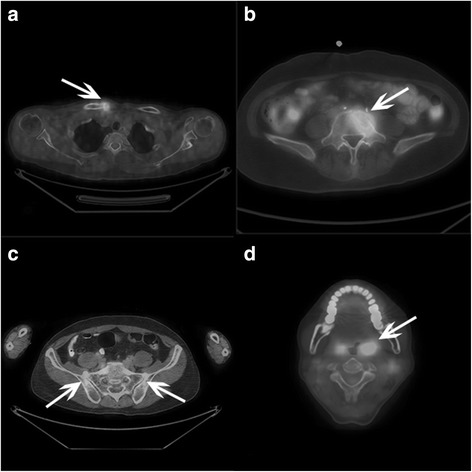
Lesions revealed by ^18^F-FDG PET/CT in SAPHO syndrome (white arrows). Abnormally increased uptake of ^18^F-FDG can be seen in a right sternoclavicular joint, b first lumbar vertebra, c bilateral sacroiliac joints, and d left tonsil
